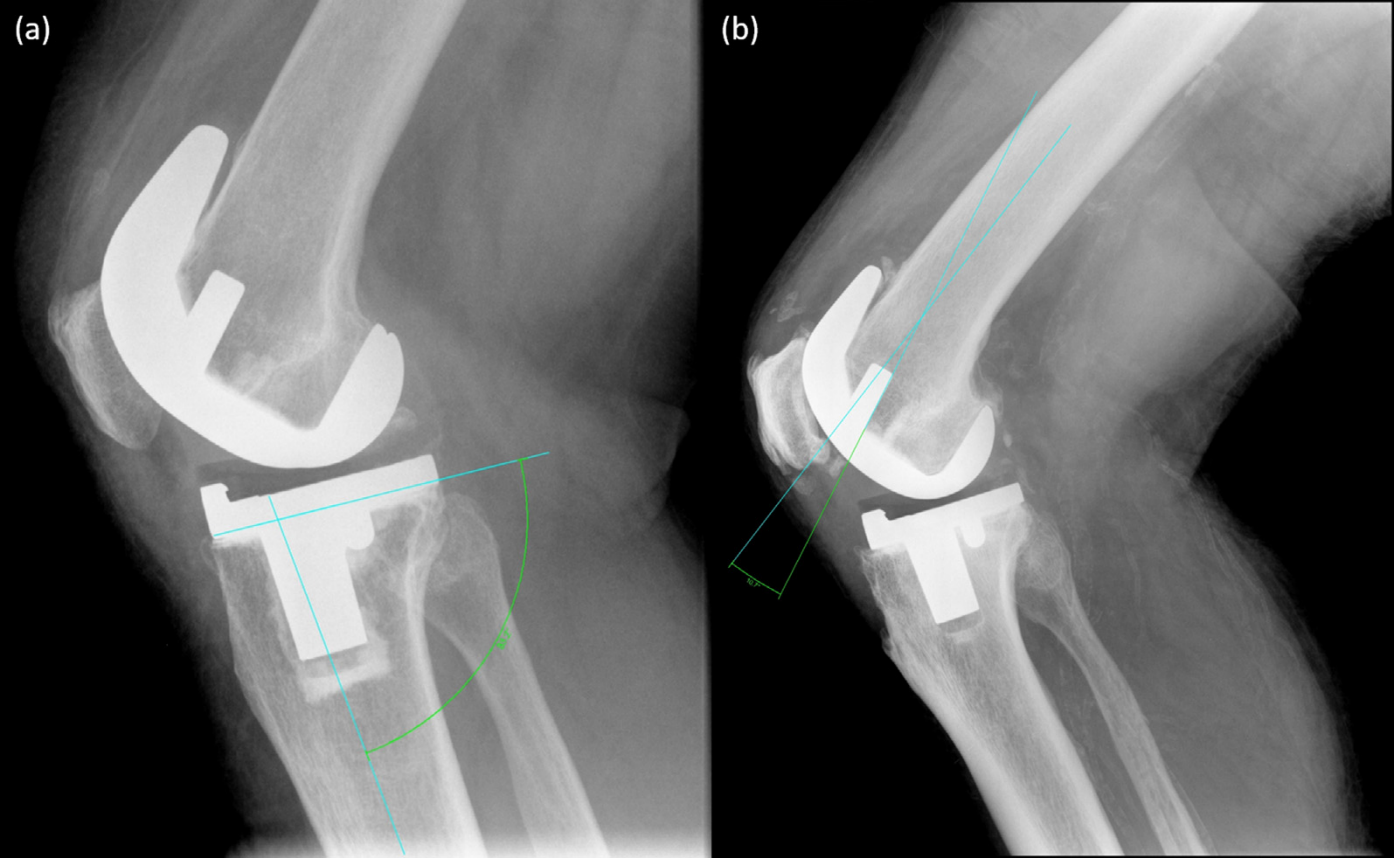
(a) Posterior tibial slope angle was measured from clinical plane X-Rays at the intersection of a line drawn across the tibial plateau and a line drawn down the middle of the tibial shaft joining two centre-points at 10 and 20 cm; (b) The flexion angle of the femur was defined as the angle between the back of the anterior flange of the femoral component, and a line drawn along the middle of the femoral shaft on the lateral knee x-ray.
